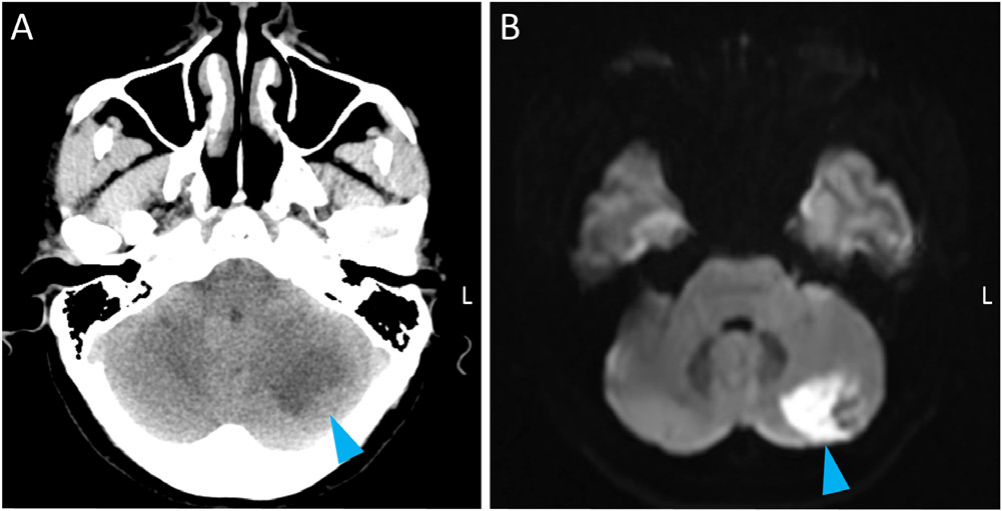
CT and MRI findings of acute left cerebellar infarction.
